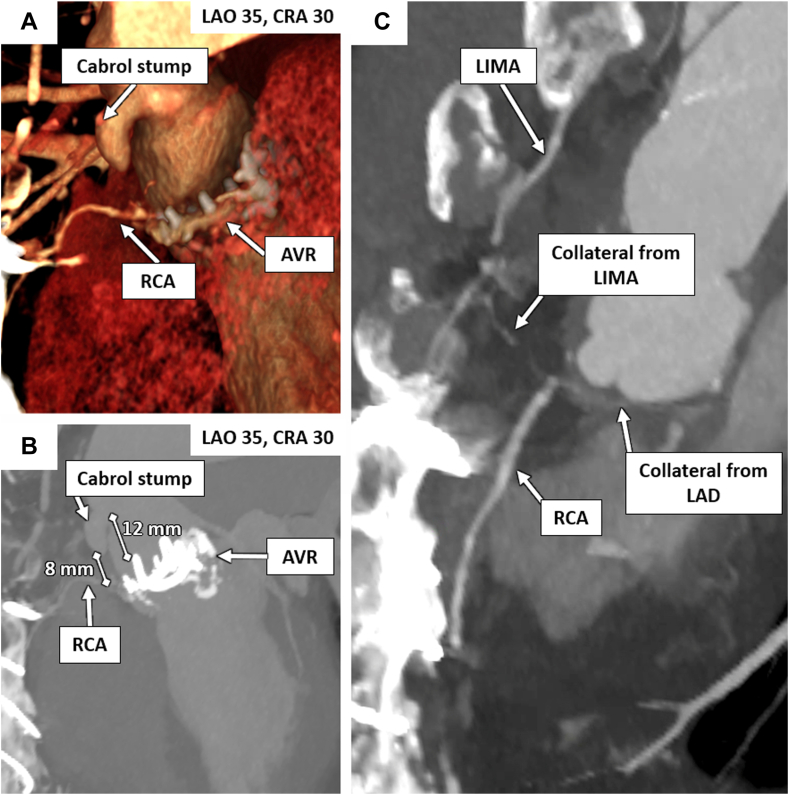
Coronary computed tomography reconstruction demonstrating the Cabrol graft stump, the distance to the reconstituted RCA, the left to right collaterals, and the optimal angles for visualization. AVR, aortic valve ring; CRA, cranial; LAD, left anterior descending coronary artery; LAO, left anterior oblique; LIMA, left internal mammary artery; RCA, right coronary artery.
